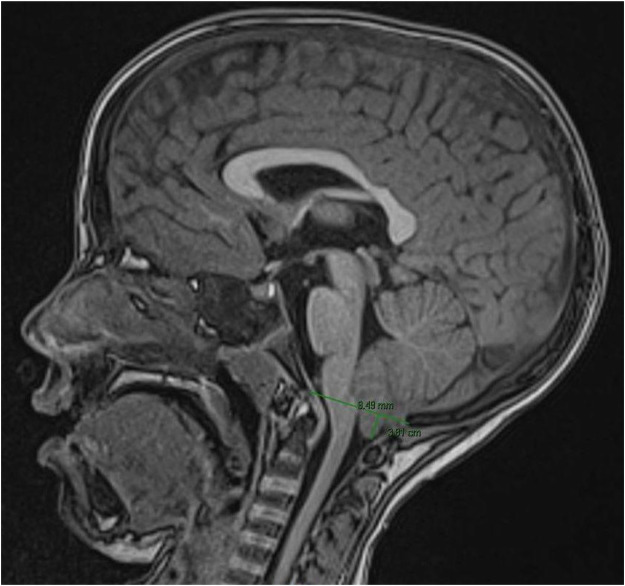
MRI at 8 years showing a type 1 Arnold-Chiari malformation, specifically a 9 mm herniation of the cerebellar tonsils beyond the foramen magnum.
